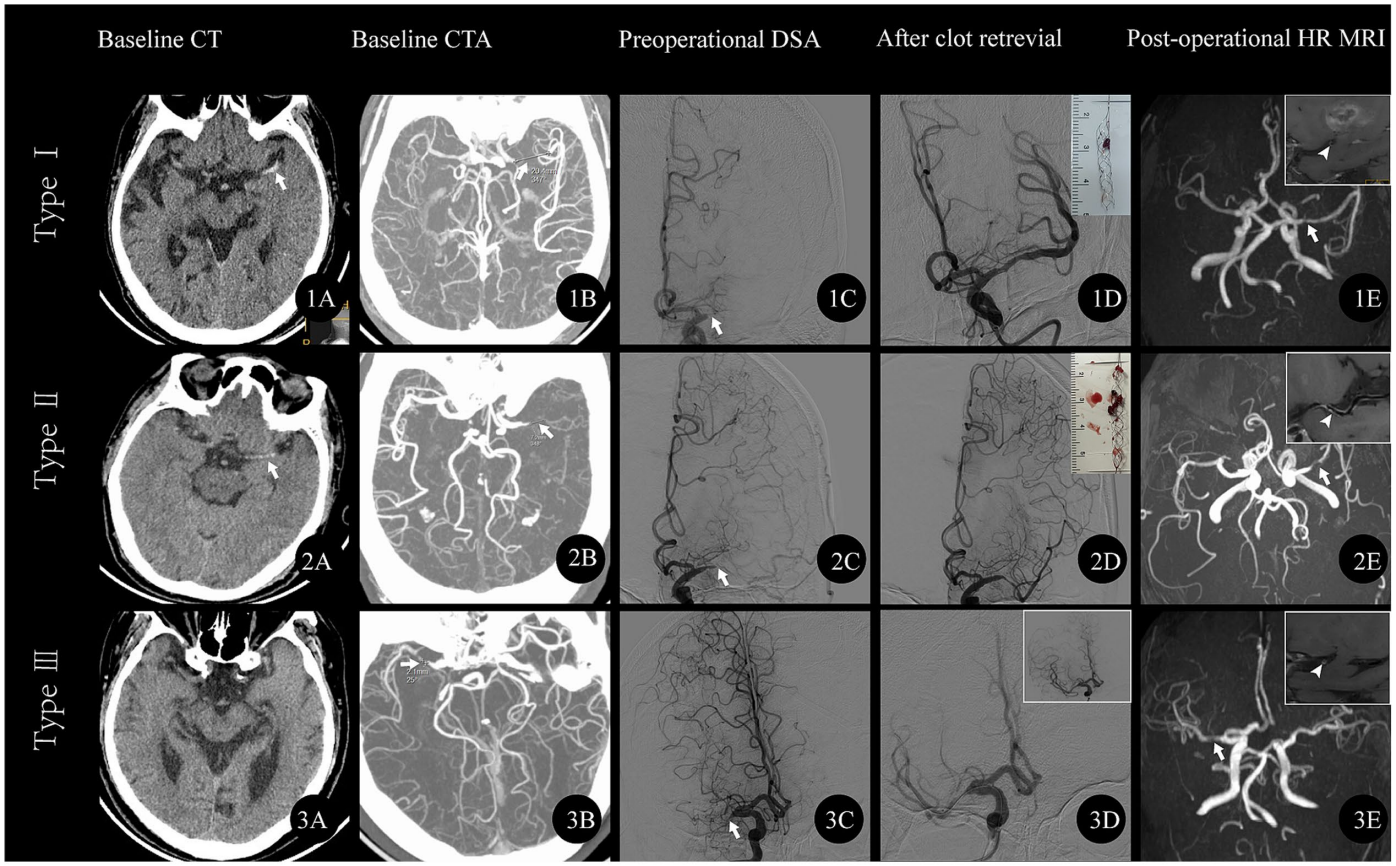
Representative cases of respective TS subtype. (A) TS-type I A middle-aged male patient presented with sudden right limb weakness lasting 19 h, muscle strength grade 0, left eye deviation, and NIHSS score of 17. (1A) Baseline CT shows HAS in the left MCA (arrow); (1B) CTA reveals left MCA occlusion with an occlusion length of 14 mm; (1C) Angiogram confirms left MCA occlusion (arrow); (1D) Post-clot retrieval angiogram shows mild stenosis in the left MCA; the thrombus is visualized in the upper right frame; (1E) Follow-up MRA shows mild stenosis in the left MCA (arrow). The high-resolution MR (TIWI) image in the upper right frame reveals an irregular plaque within the left MCA (white arrowhead). (B) TS-type II A middle-aged male patient presented with sudden right limb weakness lasting 8 h, muscle strength grade 4, and NIHSS score of 3. (2A) Baseline CT shows HAS in the left MCA (arrow); (2B) CTA reveals left MCA occlusion with an occlusion length of 7.2 mm; (2C) Angiogram confirms left MCA occlusion (arrow); (2D) Post-clot retrieval angiogram shows severe stenosis in the left MCA; the thrombus is visualized in the upper right frame; (2E) Follow-up MRA shows severe stenosis in the left MCA (arrow). The high-resolution MR (TIWI) image in the upper right frame reveals an irregular plaque within the left MCA (white arrowhead). (C) TS-type III An elderly patient presented with sudden left limb weakness lasting 2 h, muscle strength grade 4, and NIHSS score of 5. (3A) Baseline CT is normal; (3B) CTA reveals right MCA occlusion with an occlusion length of 2.1 mm; (3C) Angiogram confirms right MCA occlusion (arrow); (3D) Post-clot retrieval angiogram shows severe stenosis in the right MCA without thrombus. A subsequent angiogram (upper right frame) shows mild stenosis in the right MCA; (3E) Follow-up MRA shows mild stenosis in the right MCA (arrow). The high-resolution MR (TIWI) image in the upper right frame reveals an irregular plaque. NIHSS, National Institutes of Health Stroke Scale; HAS, hyperdense artery sign; MCA, middle cerebral artery; CTA, CT angiography; MRA, Magnetic Resonance Angiography.
